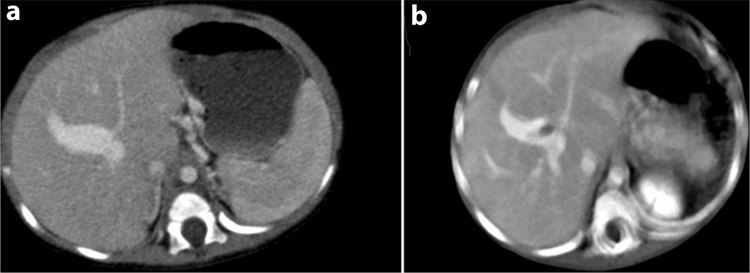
a, b. Preoperative axial computed tomography showing fusiform portal venous aneurysmal dilatation (a), corresponding postoperative axial computed tomography image (b) demonstrates thrombus inside this dilated portal vein.
